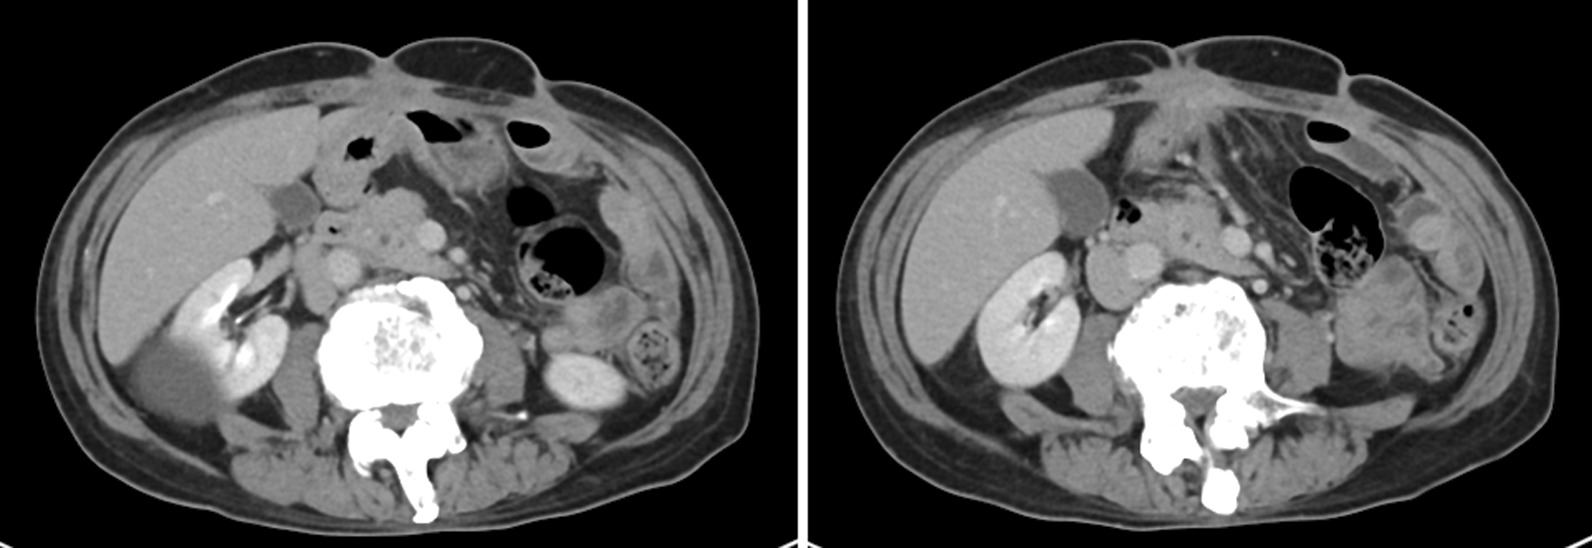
Contrast-enhanced computed tomography scan obtained after six courses of the SOX regimen showing significant reduction in the diameter of the abdominal wall invasion and metastatic lymph nodes. SOX regimen: chemotherapeutic regimen comprising S-1 (120 mg/day) and oxaliplatin (100 mg/m^2^)
